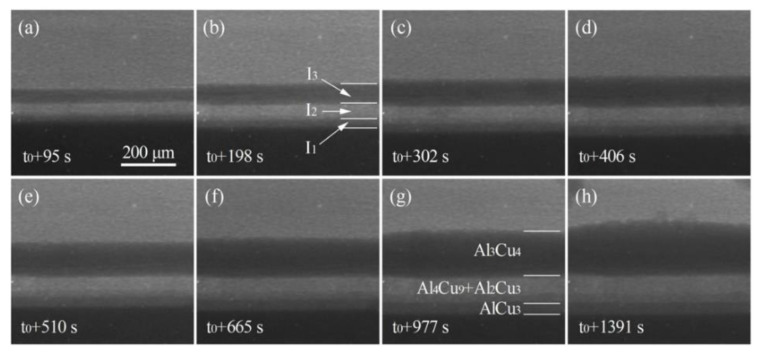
Sequence of in situ radiographs (a–h) showing the growth of IMCs layers (*t*_0_ is assigned to the onset of colling).
